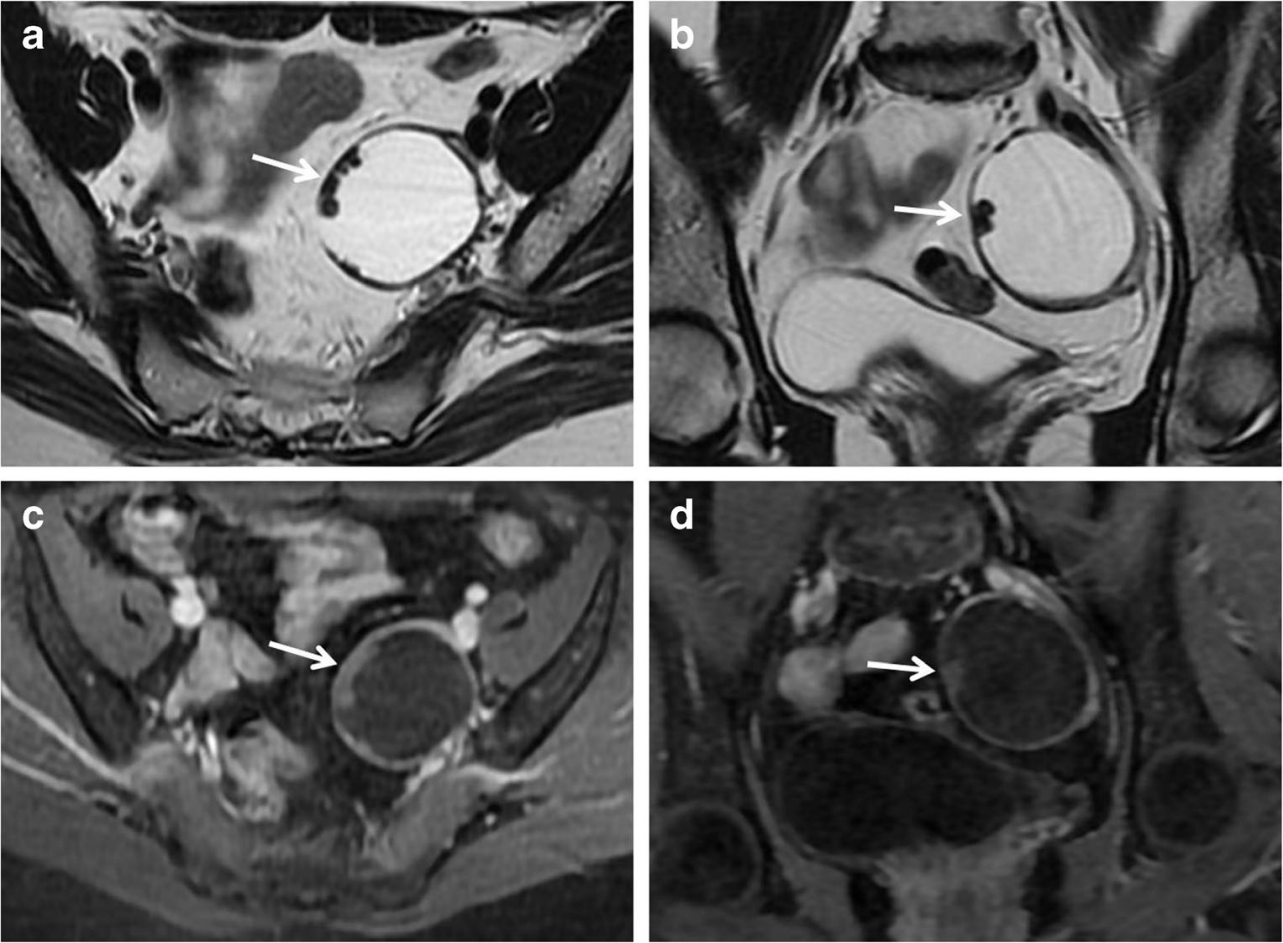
Cystadenofibroma in a 54-year-old woman. (a) Axial and (b) coronal T2-weighted images show a predominantly cystic mass with small areas of fibrous stroma along the wall characterized by homogeneous low signal intensity (*white arrows*). (c) Axial and (d) coronal contrast-enhanced fat-suppressed T1-weighted images show minimum enhancement of the cystic wall and of the fibrous component (*white arrows*)
